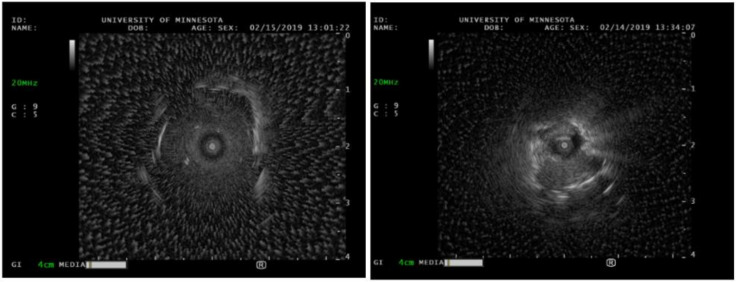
Radial EBUS ultrasound images of a peripheral nodule. The left image is consistent with the concentric signal, indicating that the radial EBUS probe is within the target. The right image is consistent with the eccentric signal, indicating that the radial EBUS probe is tangential to the target.
